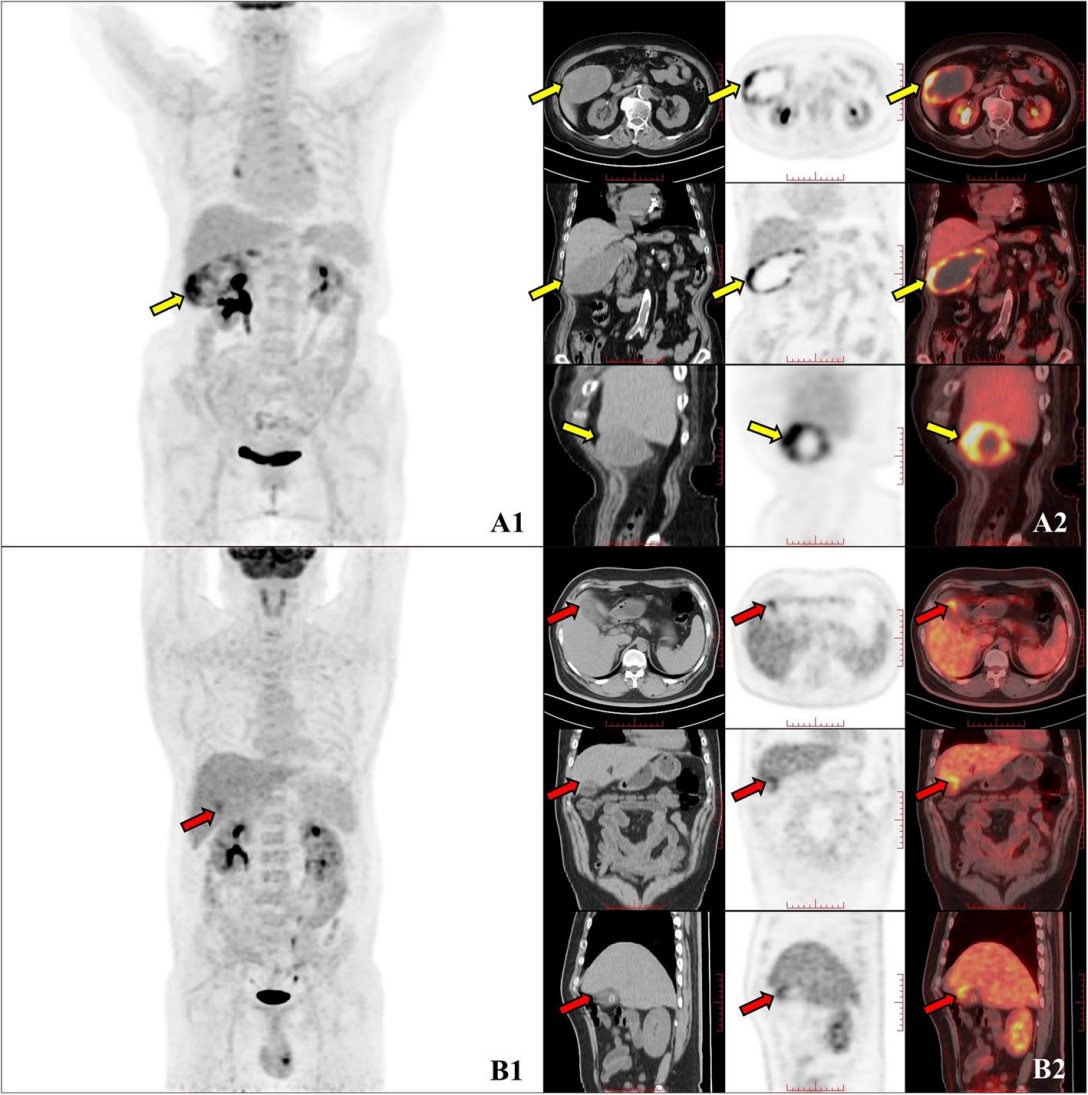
Images A1 and A2 show a 77-year-old woman with medium-differentiated gallbladder adenocarcinoma (yellow arrow). During the physical examination one month prior, the patient was found to have a space-occupying gallbladder, without jaundice, fever, abdominal pain or abdominal mass. The patient had no history of cholecystolithiasis, gallbladder polyps or cholecystitis. The CEA was 6.59, and the CA19-9 was 9.22. The lesion showed that SUVR was 5.5 (A1-MIP image of ^18^F-FDG PET/CT; A2-First line: axial PET, CT, and PET and CT fusion images; Second line: coronal PET, CT, and PET and CT fusion images; Third line: sagittal PET, CT, and PET and CT fusion images). Images B1 and B2 show a 39-year-old man with acute cholecystitis with chronic inflammation of the gallbladder mucosa (red arrow). The patient had no obvious cause of abdominal pain with fever for 1 month. The patient had a medical history of cholecystolithiasis. The CEA was 6.59, and the CA19-9 was 9.22. The lesion showed an SUVR of 2.1 (A1-MIP image of ^18^F-FDG PET/CT; A2-First line: axial PET, CT, and PET and CT fusion images; Second line: coronal PET, CT, and PET and CT fusion images; Third line: sagittal PET, CT, and PET and CT fusion images)
